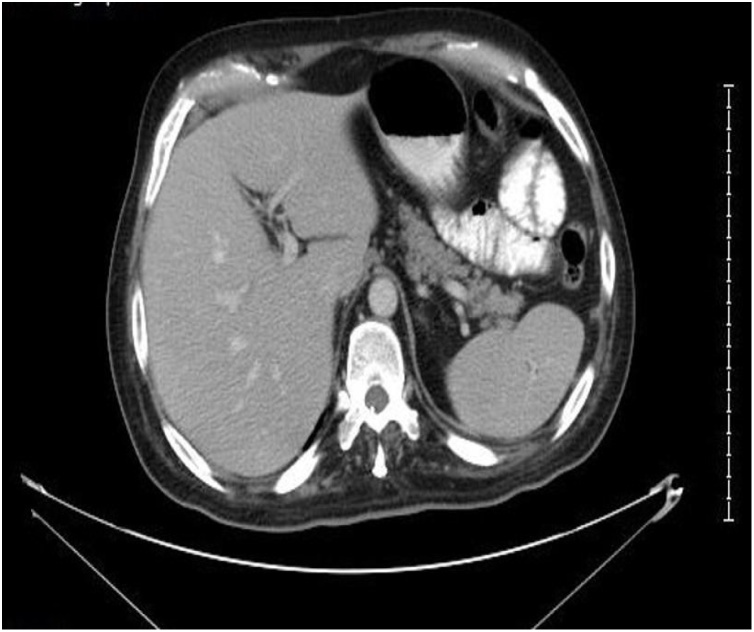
CT scan upper abdomen with IV contrast shows no abdominal organs metastases.
